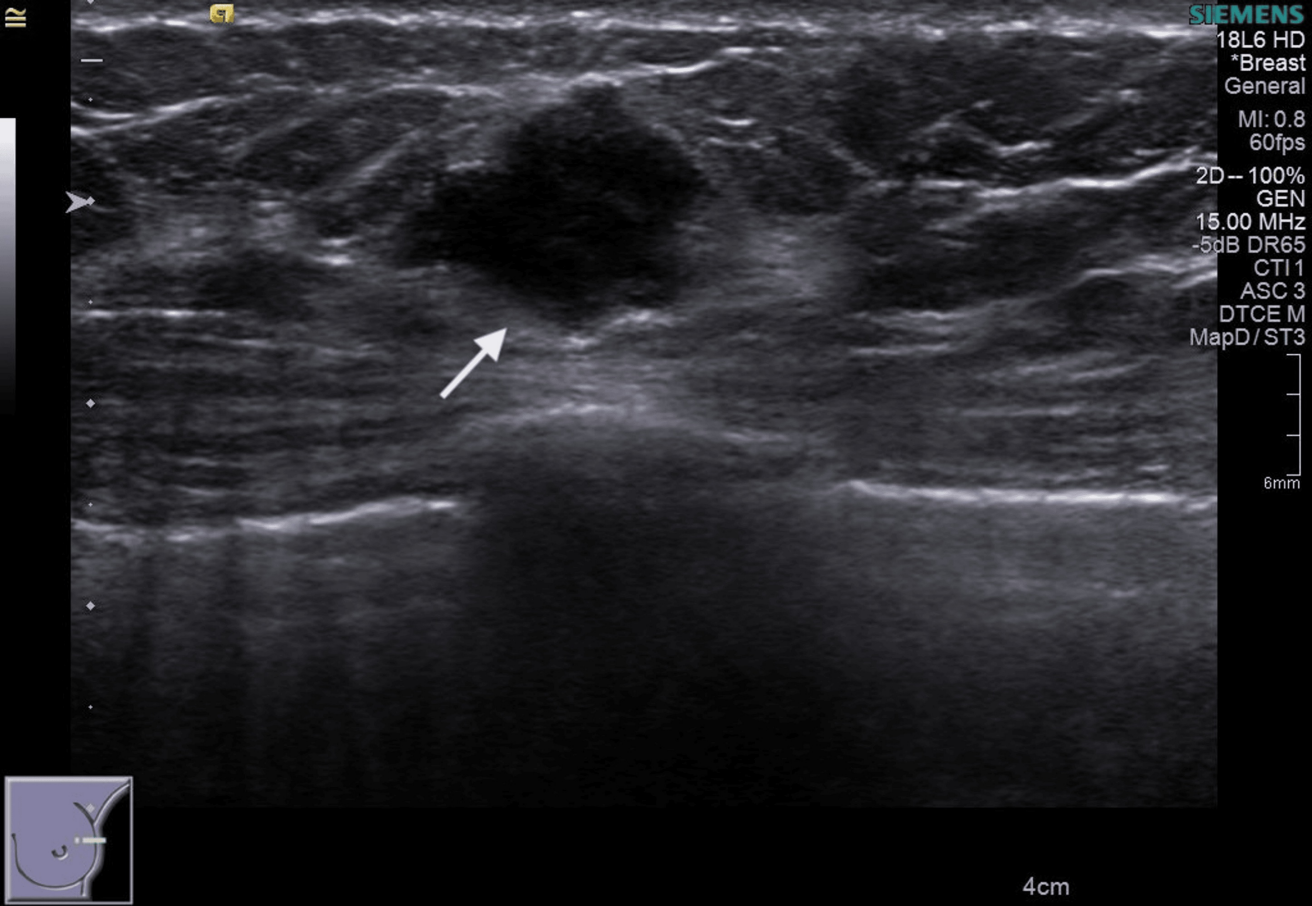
Case 3 - 57-year-old woman, left breast targeted ultrasound (US) scan of the upper pole. The arrow shows a 16 mm hypoechoic lesion.
